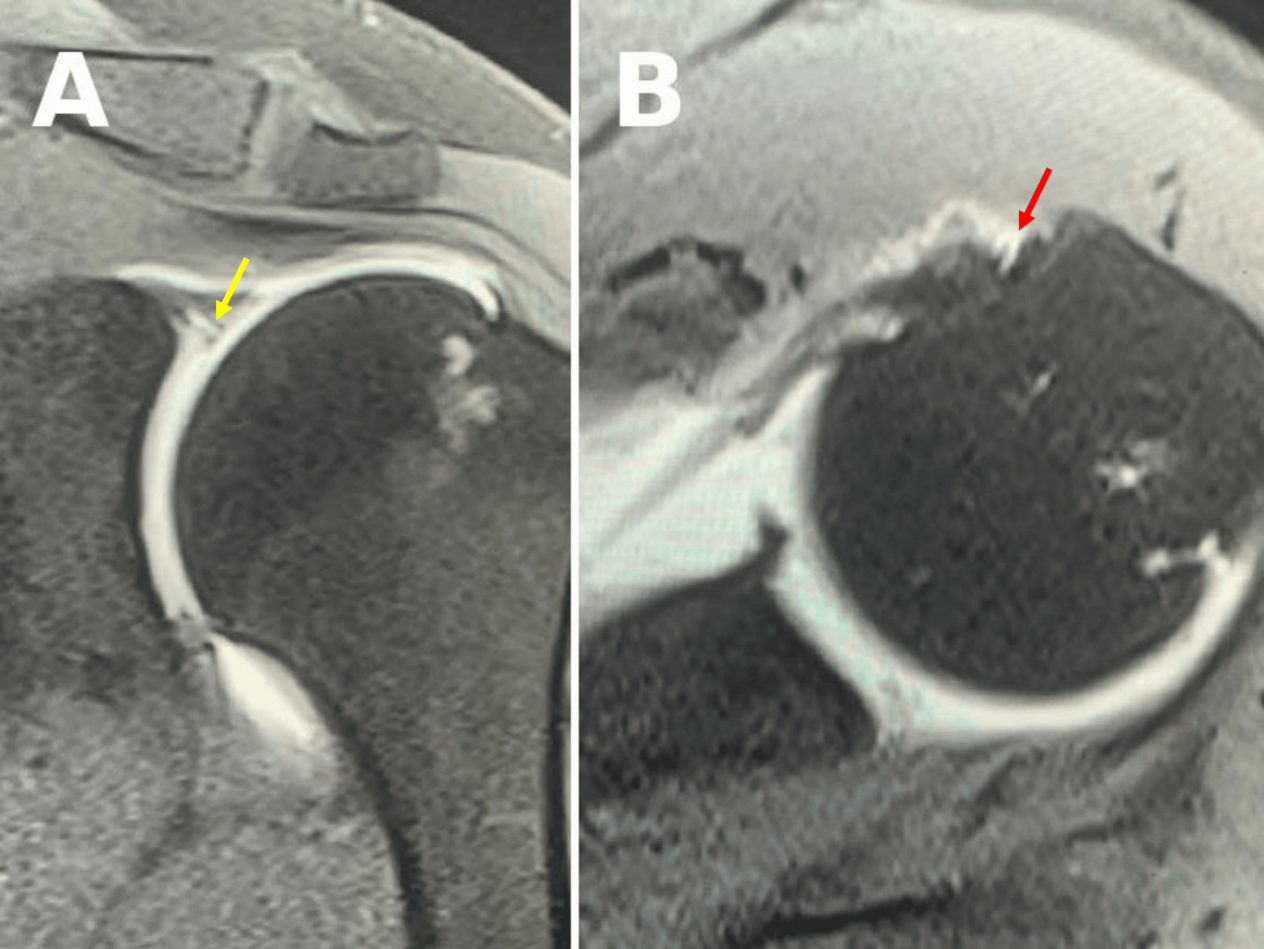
Preoperative MRA with contrast of the left shoulder showing a SLAP tear. (A) T2-weighted coronal view with a visible partial tear of the biceps (yellow arrow). (B) T2-weighted axial view showing contrast communicating with synovial bicipital groove (red arrow). MRA: magnetic resonance arthrogram, SLAP: superior labral anterior-posterior.
